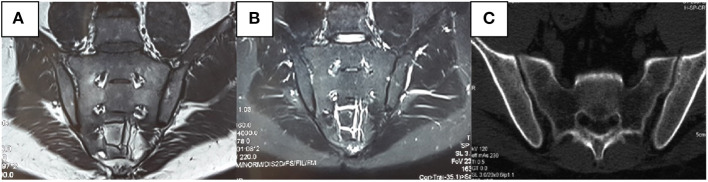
Male, 31 years old, bilateral hip pain for 8 months and lumbago for 2 months. C-reactive protein, rheumatoid factors, and human leukocyte antigen-B27 were all negative. Alkaline phosphatase was 346 U/L. Inorganic phosphorus was 0.48 mmol/L. No apparent abnormalities were observed in the sacroiliac joint (SIJ)–magnetic resonance imaging T1-weighted [T1W, (A)] and short-tau inversion recovery [STIR, (B)] sequences. It can be seen in the computerized tomography that the left SIJ is coarse rather than smooth (C).
